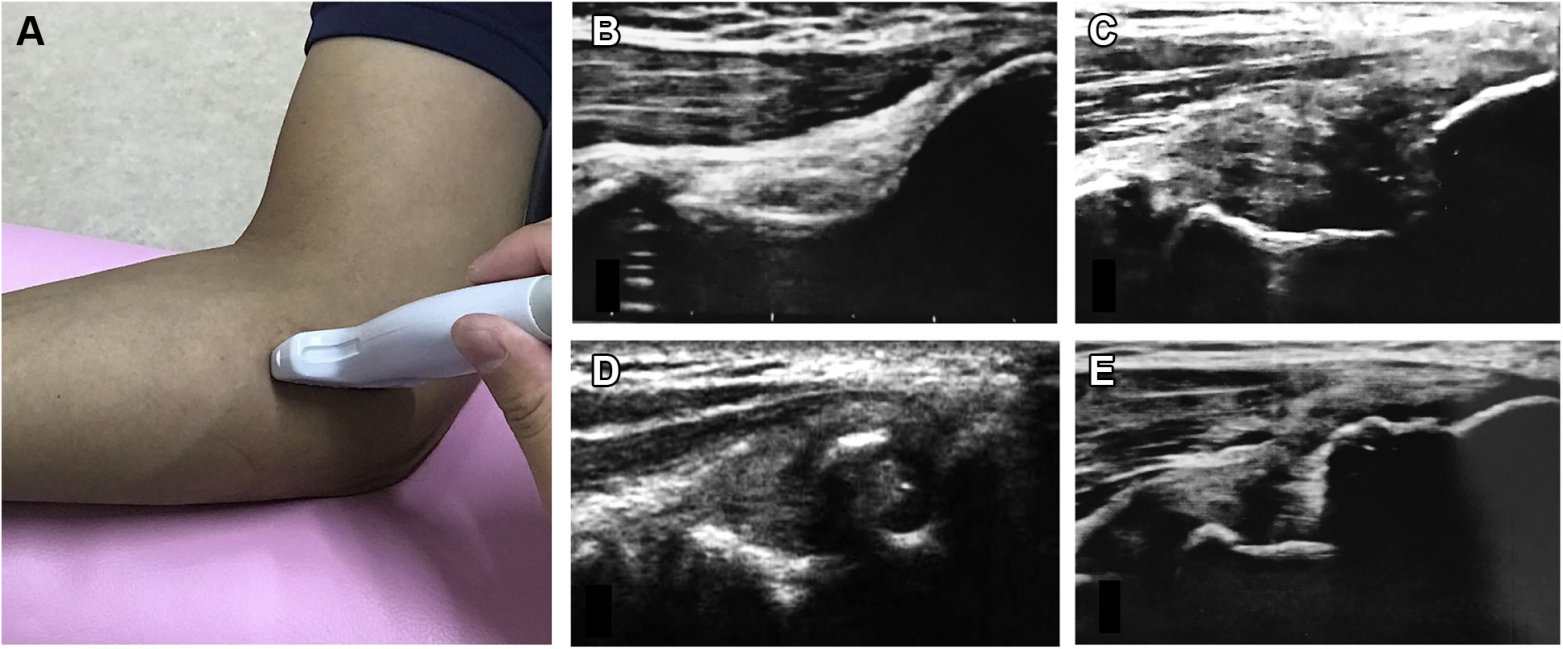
Ultrasonographic examination of the anteroinferior aspect of the medial epicondyle. To assess the medial aspect of the elbow, the elbow is flexed at 90°, and the forearm is placed in the supinated position. A linear transducer is placed on the medial aspect of the elbow to obtain an image that included the *top* of the medial epicondyle, anterior bundle of the medial ulnar collateral ligament, and sublime tubercle (A). The morphologies of the medial epicondyle are classified into four types: normal (B), irregular type (C), fragmented type (D), and hypertrophic type (E).^20^
